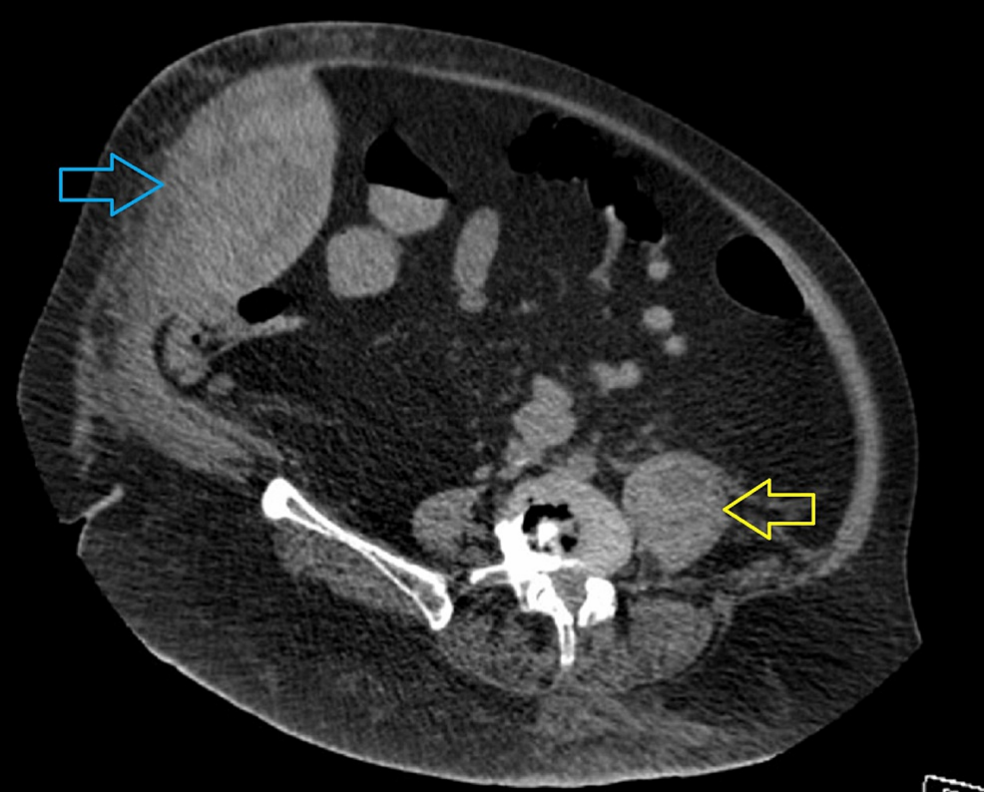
Right rectus sheath (blue arrow) and left iliopsoas (yellow arrow) hematomas on CT scan.
